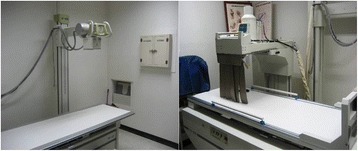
The room where simple and gastrointestinal series radiography was performed.
